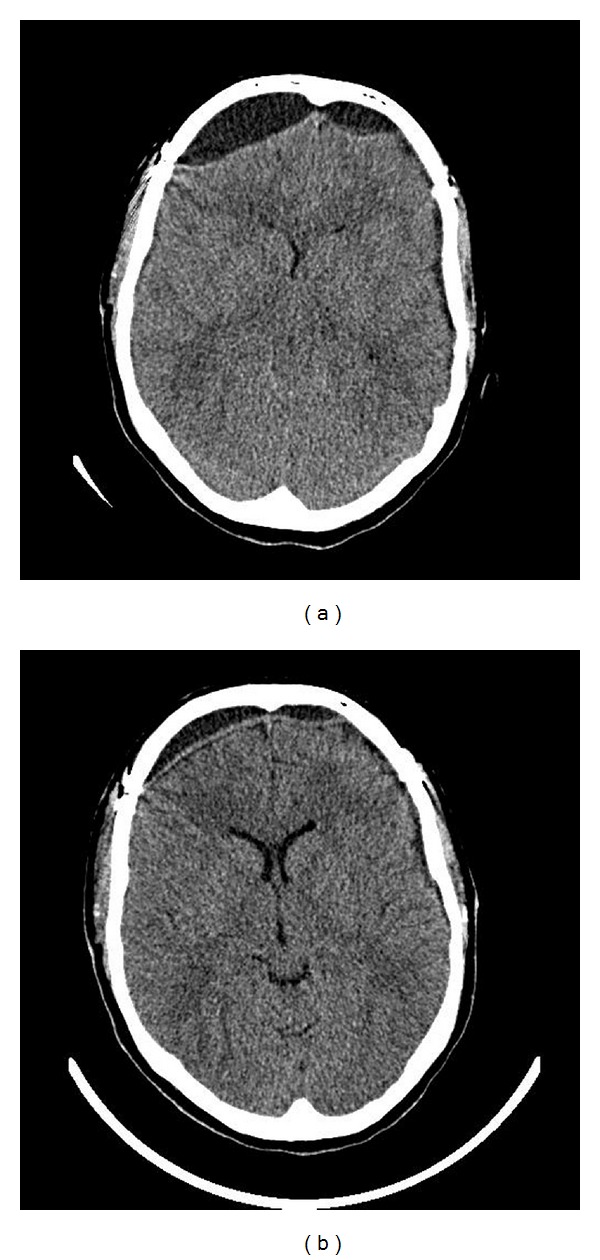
(a) Head CT of our patient the day before the EBP was performed. CSF leak resulted in large bifrontal fluid collections. (b) Head CT of the same patient the day after the EBP. The extradural CSF collection is significantly smaller in size. A possible explanation for this finding is the effect of the EBP on the intracranial pressure (as evidenced by the increased intraventricular space) prohibiting further leakage through an existent frontal tear on the dura.
